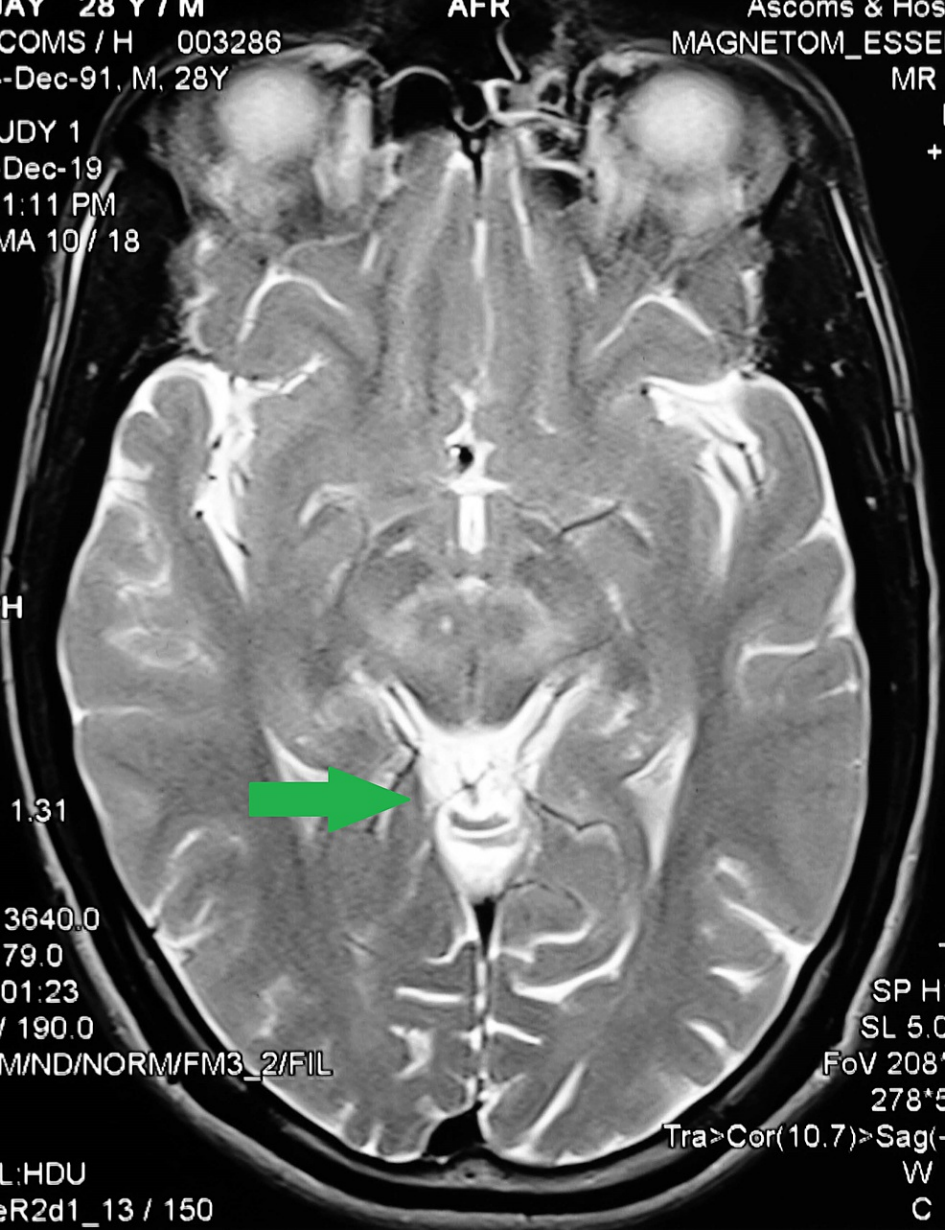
Magnetic resonance imaging (MRI) of the brain revealing T2-weighted high-signal intensities in the caudate nucleus and putamen of bilateral basal ganglia, with sparing of the globus pallidus, ventrolateral thalami, the brainstem, and pontine region, giving an appearance of “face of a giant panda” (green arrow).
